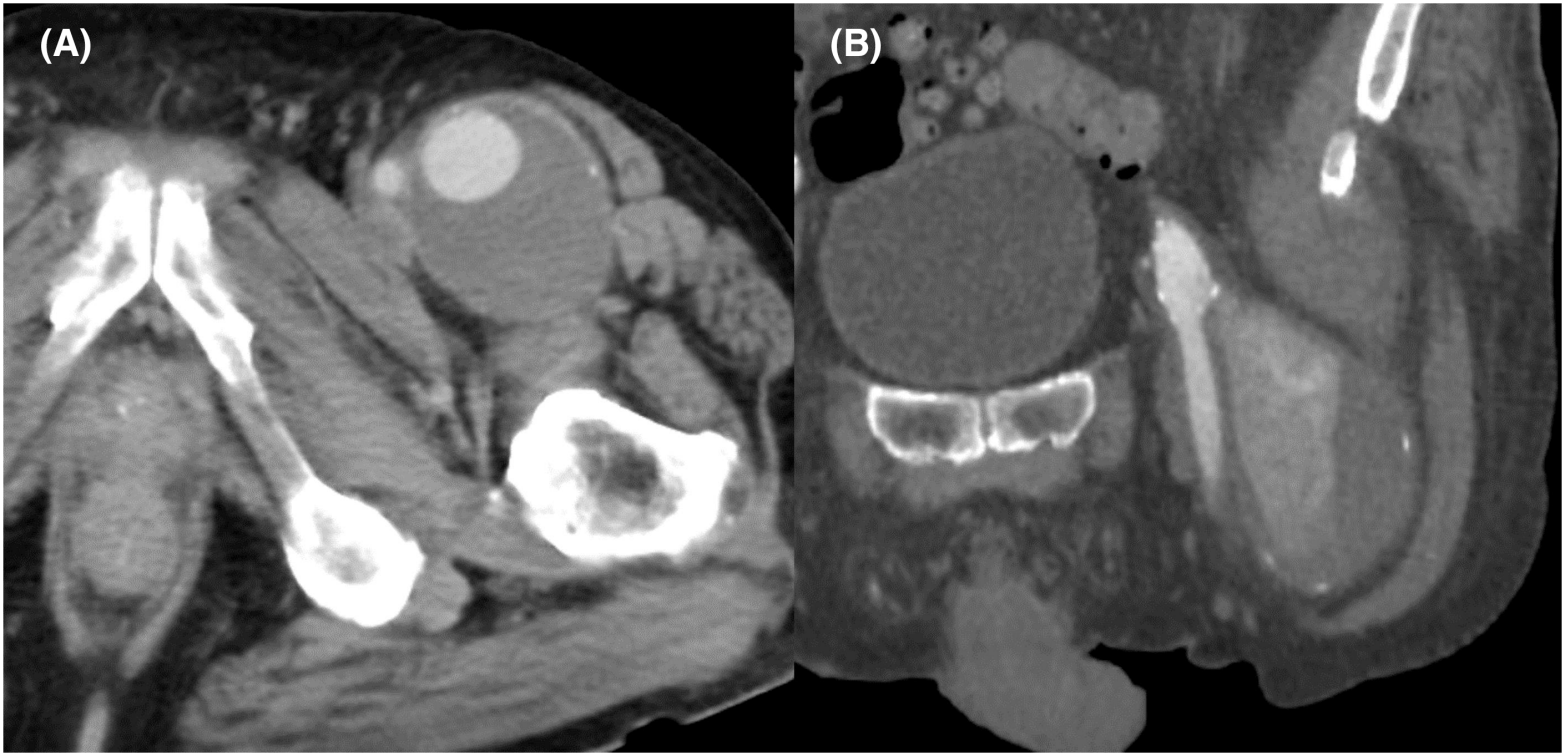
CT showed left DFAA (A) and proximal neck from bifurcation was very short (B).
